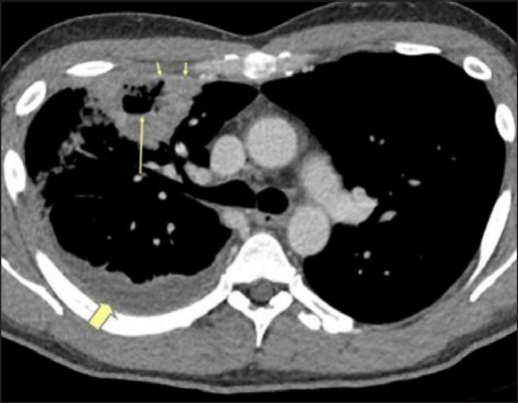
Pulmonary abscess*. A 36-year-old male was admitted with sepsis a week earlier. A CT was performed due to low response to antibiotic treatment. A large cavity is seen in the right upper lobe with an air-fluid level (long arrow). Rim enhancement is seen in the cavity wall anteriorly (short arrows). A pleural effusion is also seen (thick arrow).
